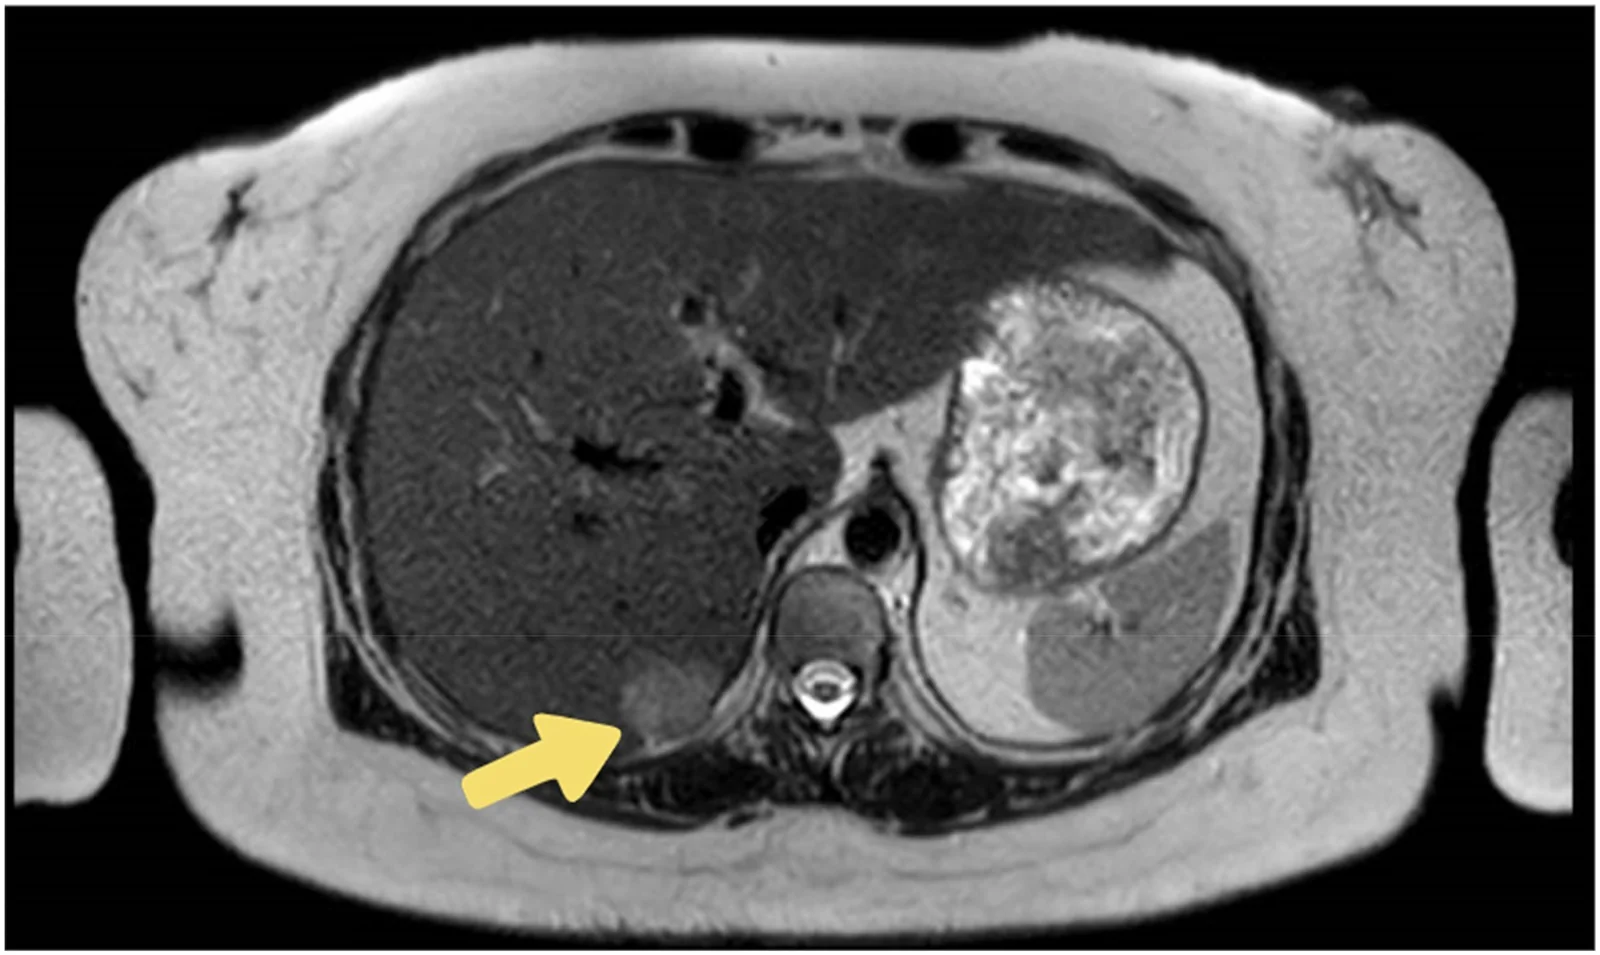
Magnetic resonance imaging (MRI) of the liver demonstrating metastatic lesion in segment VII. Axial image shows a right hepatic lobe mass in segment VII (yellow arrow), measuring approximately 2.2 × 3.2 × 3.1 cm (previously 2.0 × 2.5 cm), with early irregular enhancement and absence of contrast uptake on the hepatobiliary phase, findings suggestive of metastasis in a patient with known adrenocortical carcinoma.
